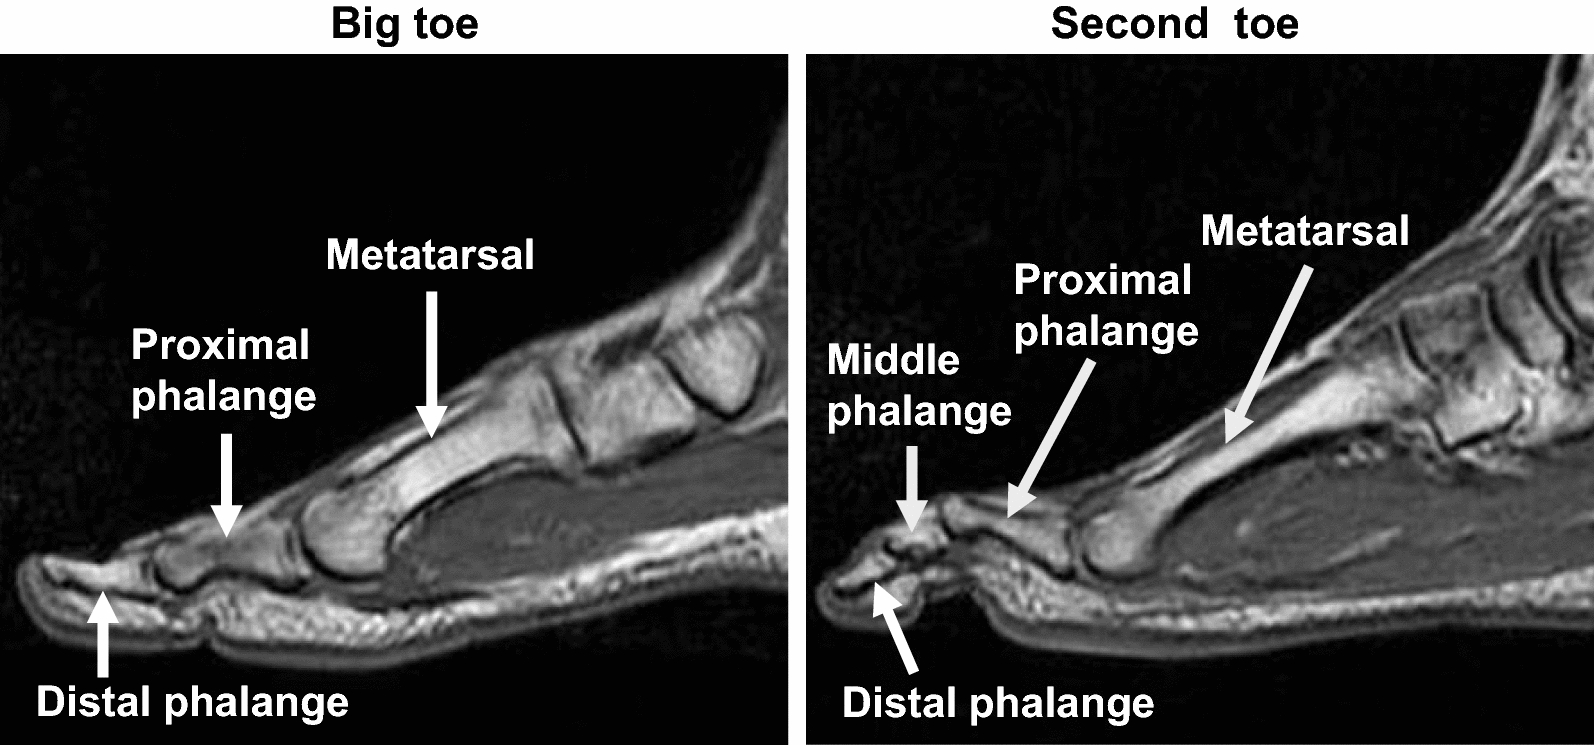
Representative magnetic resonance imaging scans used for measuring the lengths of the forefoot bones of the big and second toes. The forefoot bones of the big toe included the distal phalanx, proximal phalanx, and metatarsal bones. The forefoot bones of the second toe included the distal phalanx, middle phalanx, proximal phalanx, and metatarsal bones
